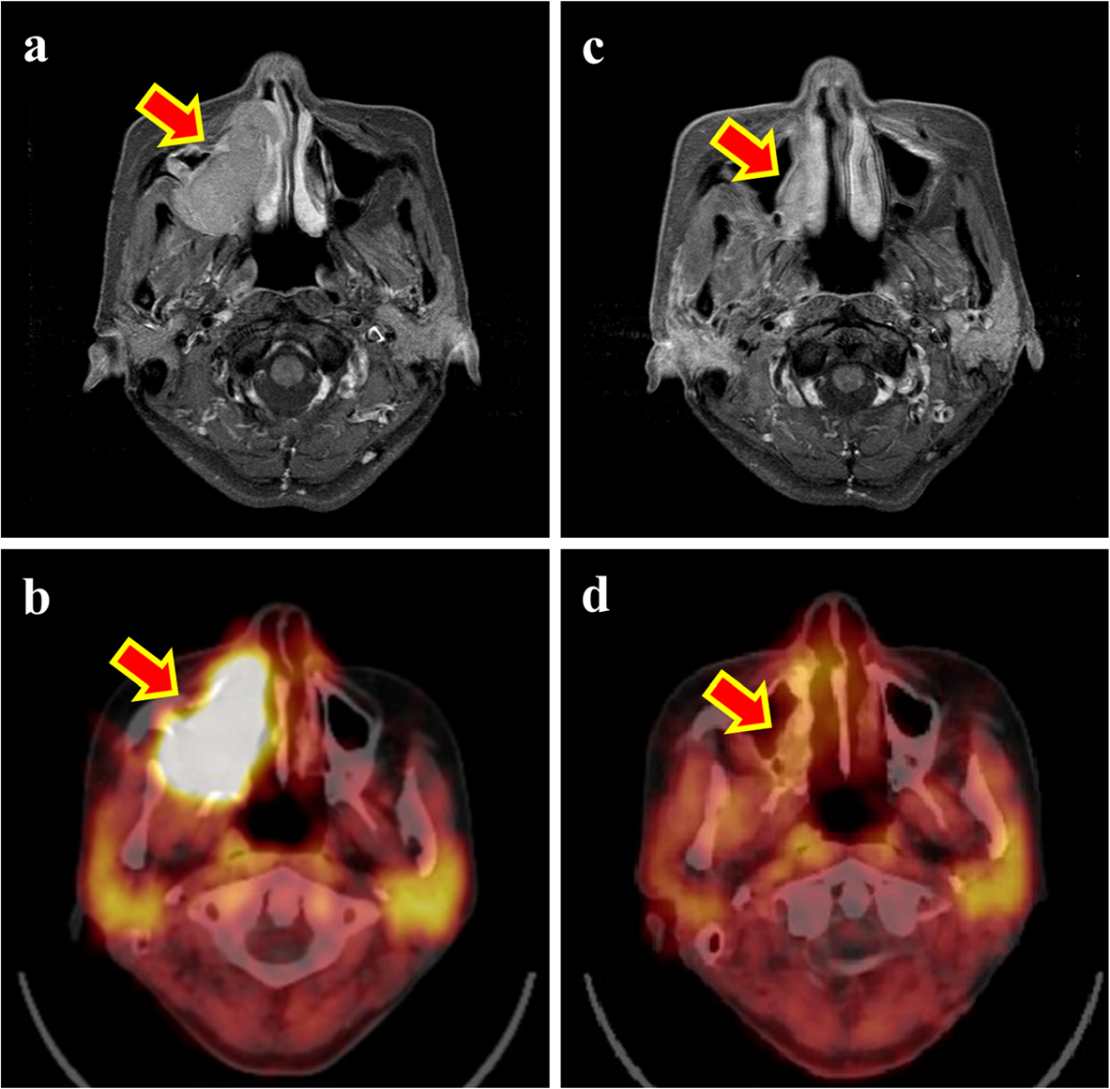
A 49-year-old female who received 57.6 GyE for paranasal sinus cancer. The arrows indicate the tumors. a. Magnetic resonance imaging (MRI) showed a 53 × 44 mm mass in the paranasal sinus region. b. A methionine (MET)-PET/CT image prior to treatment demonstrated a high accumulation mass in the region shown in the MRI. c. MRI revealed tumor shrinkage 44 days after carbon ion radiotherapy. d. The MET-PET/CT image after the treatment demonstrated decreased MET uptake.
